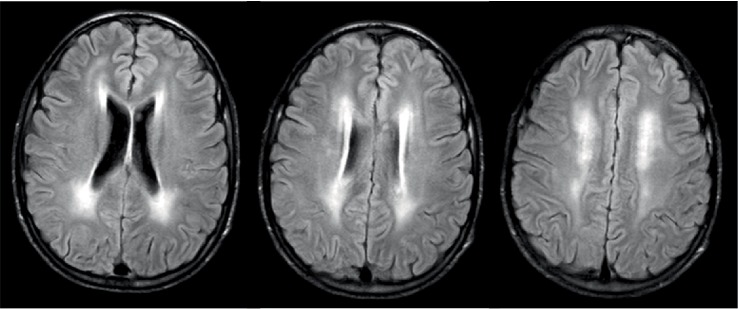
Brain MRI. Axial FLAIR images show symmetric and confluent increase signal intensity in deep white matter.
